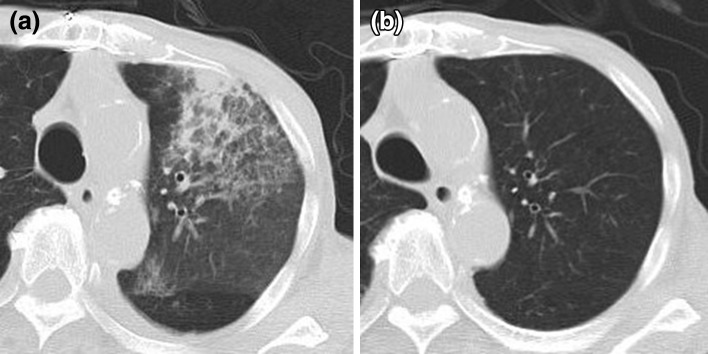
CT scan at the level of the upper lobe in July 2011. a Consolidation in the left upper lobe is apparent. b The CT scan performed 4 months later at the same level shows resolution of the consolidation
